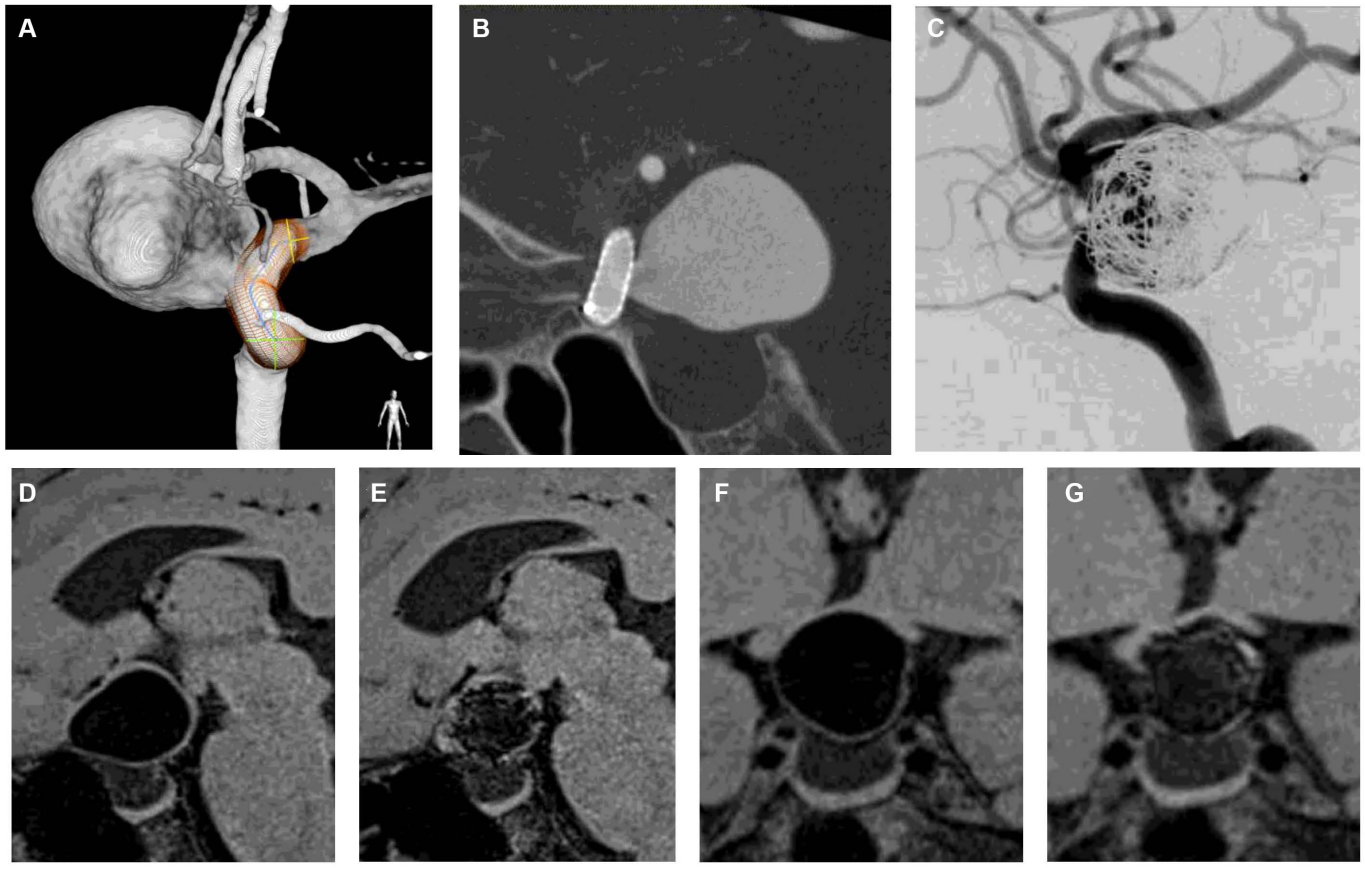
Representative case of aneurysm shrinkage after flow diversion with loose coil embolization. (A) is a 3D rotational angiogram of the aneurysm. The aneurysm was located in the paraclinoid portion with a maximum diameter of 23 mm. The aneurysm was compressing the optic nerve, and the patient had visual field defects. (B) is a corn beam CT after flow diverter implantation. (C) shows DSA after adjunctive coil embolization with a VER of 6.1%. (D,F) are DANTE T1-SPACE before treatment, and (F,G) are DANTE at 12 months after treatment. Twelve months after treatment, the aneurysm was shrunk (24% reduction rate), indicating that the optic nerve compression was relieved (D–G). DSA 12 months after treatment showed that the aneurysm was completely occluded, and the patient’s visual field defects had improved. DANTE T1-SPACE has high artifact immunity and allows easy visualization of the aneurysmal margins even when the coil is implanted within the aneurysm (E,G).
